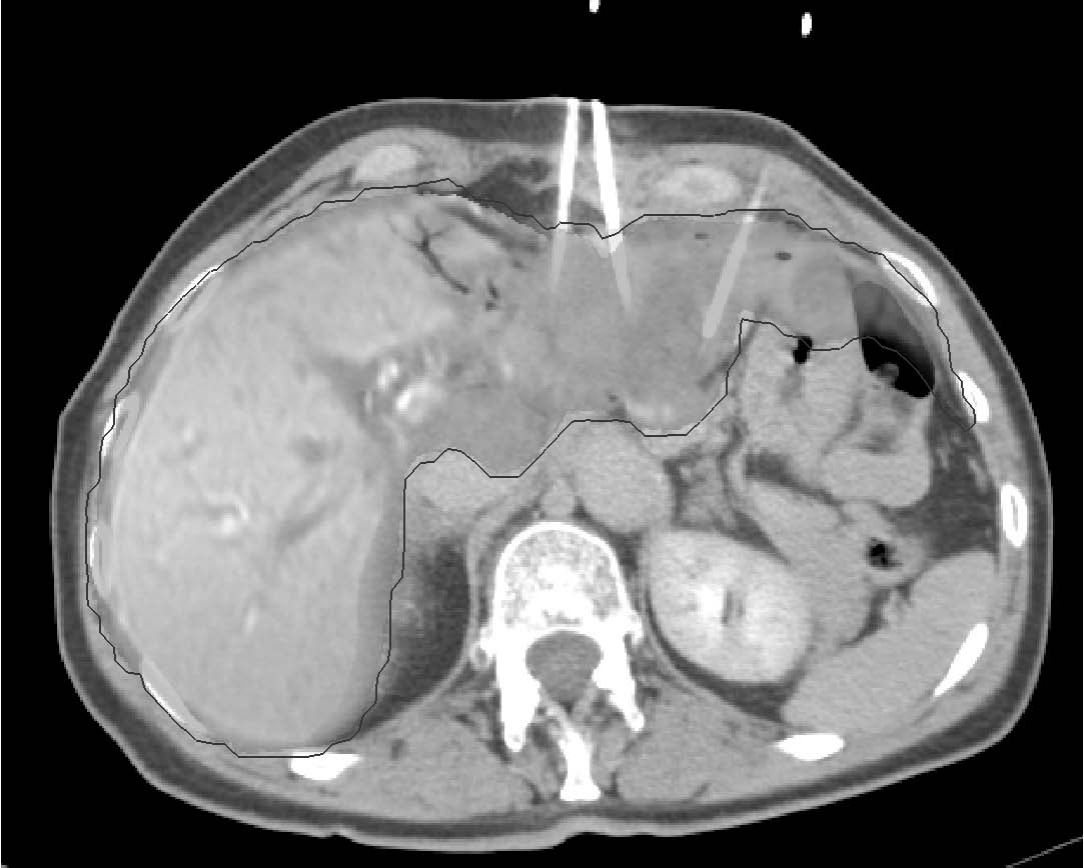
Image-fusion: Contrast-enhanced computed tomography (CT) after CT-guided positioning of brachytherapy catheters (arrows) in a liver metastasis of a colorectal carcinoma, merged with the last magnetic resonance imaging of the liver acquired after all interventions (grey delineation). The hypointensity area shows the impairment of hepatocyte function in the left liver lobe.
